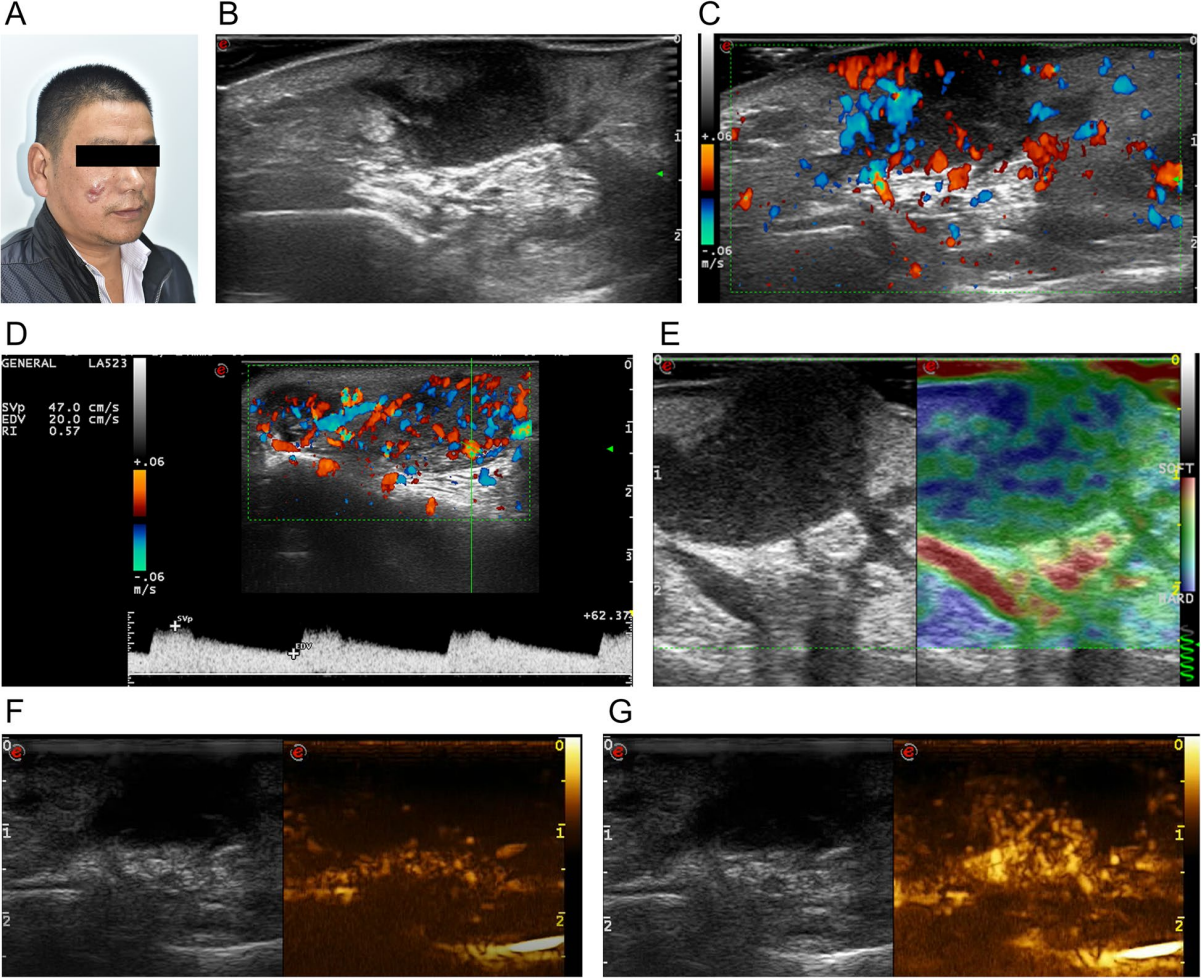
A DFSP with three nodules in the right face of a 48-year-old man. B Transverse ultrasonogram revealing an ill-defined, hypoechoic with finger-like projections and posterior hyperechoic area, heterogeneous, and subcutaneous lesion extending from the skin to bone. C Color Doppler ultrasonogram revealing intralesional and peripheral vascularity and rich vascularization with vascular densities reaching 4.5/cm^2^. D Power Doppler ultrasound revealed artery with peak systolic blood flow velocity of 47.0 cm/s and resistance index of 0.57. E Elastography showing an elasticity score of 3. F Contrast-enhanced ultrasound revealed the trend that contrast agent enters (the 26th second after the injection of contrast agent) the lesion from the bottom to the center. G Contrast-enhanced ultrasound revealed heterogeneous hyper-enhancement at peak (the 50th second after the injection of contrast agent), with the maximum diameter and depth of 4.3 cm and 2.1 cm respectively
